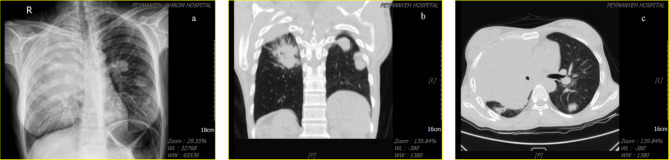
(a) Posteroanterior Chest X-ray, (b) coronal view of spiral chest CT, (c) axial view of spiral chest CT depicting multiple hemorrhagic cannonball lesions in lungs
